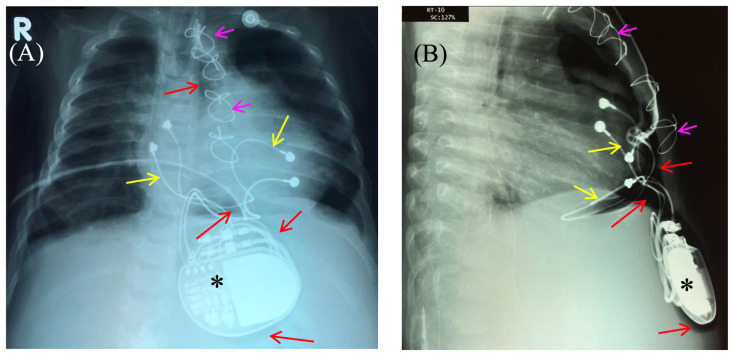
Chest X-ray—frontal (A) and lateral (B) view. Chest X-ray shows no pneumothorax, but pneumopericardium, pneumomediastinum and air in the pacemaker pocket site (red arrow) are present. One can also see the pacemaker electrodes (yellow arrow), the pulse generator (black asterisk), and the wire ligature (pink arrow). R: right side.
